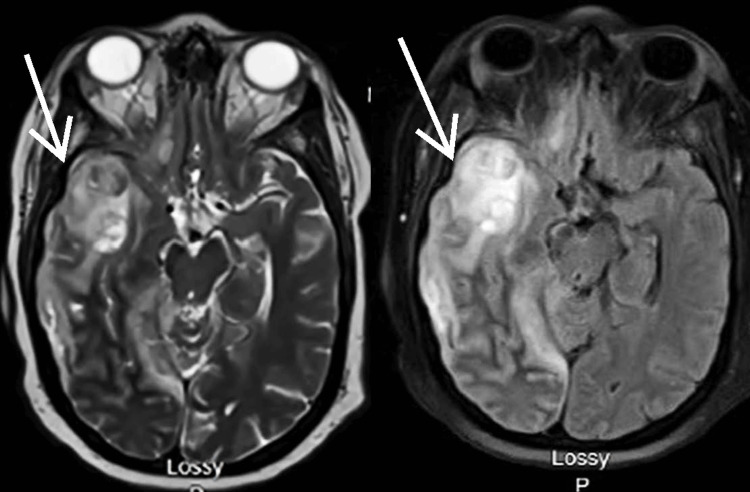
FLAIR sequence brain MRI, demonstrating the right temporal lobe hemorrhagic infarct with avid peripheral enhancement. FLAIR: fluid-attenuated inversion recovery.
